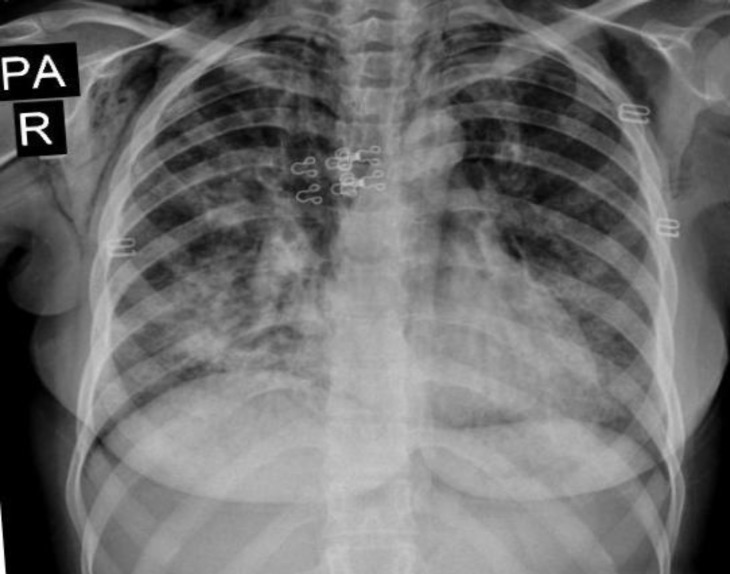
Chest radiograph showing bilateral interstitial and reticulonular changes
